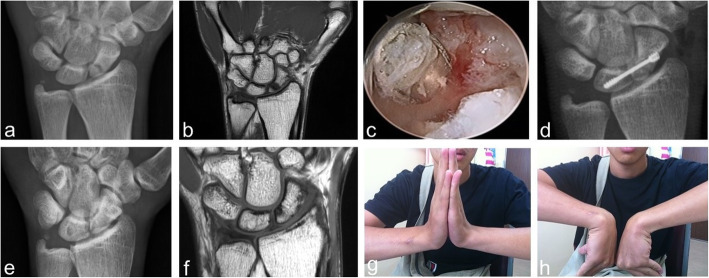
A 20-year-old male. (Case 17) (a) and (b) Radiograph and MRI 7 months after the fracture event showing the left proximal scaphoid nonunion. (c) Arthroscopic bone grafting. (d) Radiographs at the postoperative 1-month follow-up. (e) and (f) Radiograph and MRI at postoperative 7 years, revealing a solid union. (The screw was removed 8 months after surgery). (g) and (h) The patient regained good range of motion of the wrist
